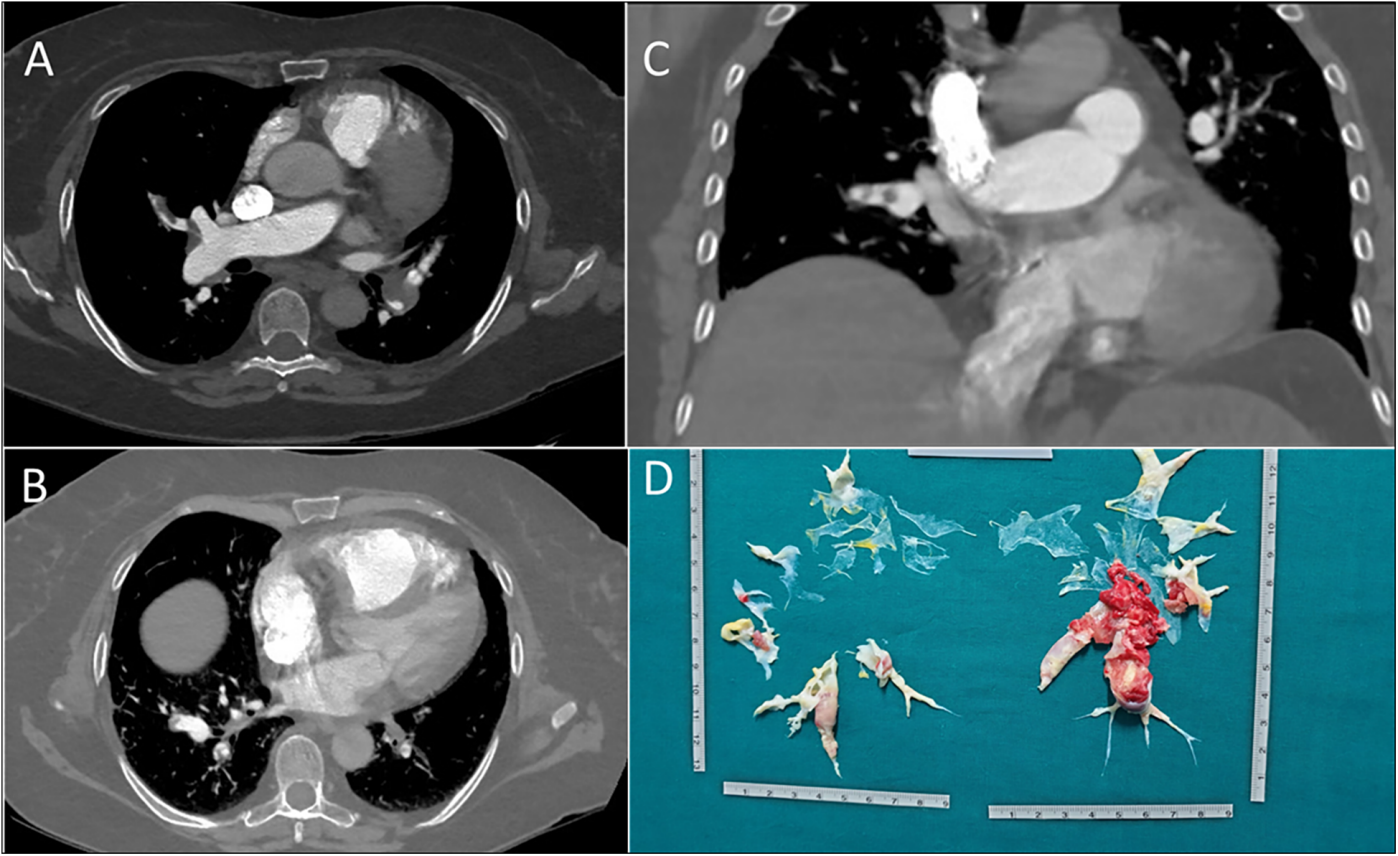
(A) axial scansion of chest CT with contrast. It shows a filling defect of a thrombo-embolic nature in the branch of the right pulmonary artery tributary to the middle lobe. (B) Axial scansion of chest CT with contrast. A filling defect of thrombo-embolic nature in the right and left pulmonary artery branches tributary to the lower lobes can be observed. (C) Coronal scansion of chest CT with contrast. It shows a filling defect of a thrombo-embolic nature in the branch of the right pulmonary artery tributary to the middle lobe. (D) Postoperative surgical finding of pulmonary thromboendarterectomy.
